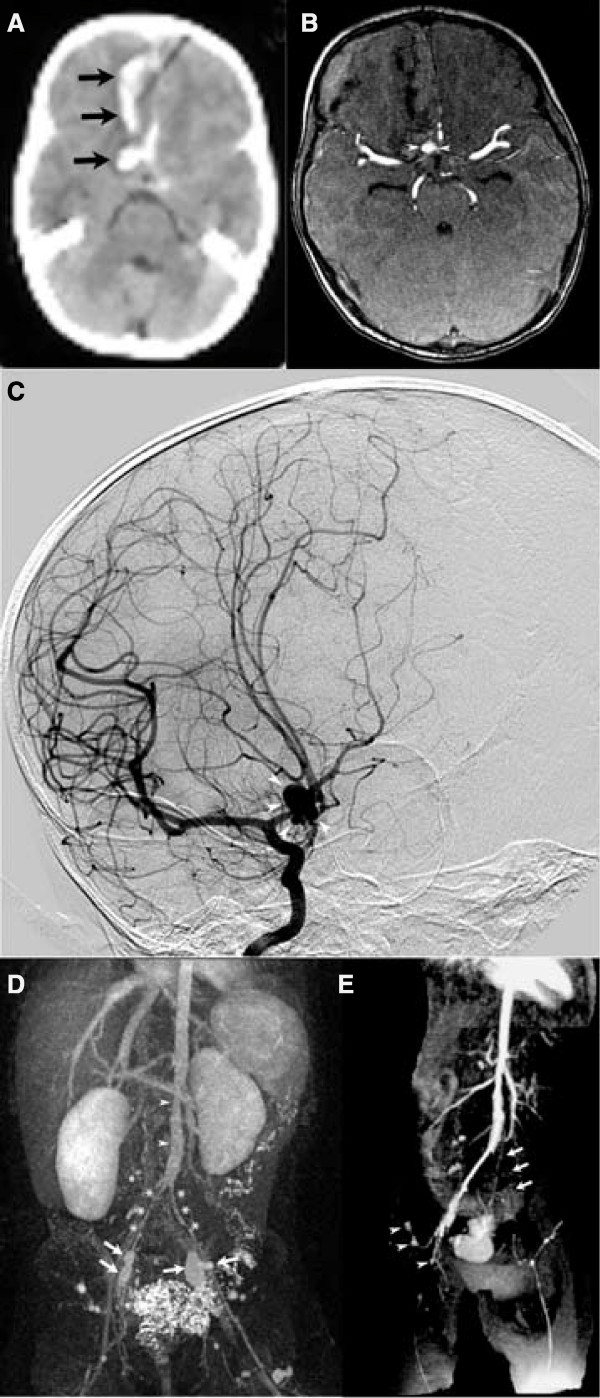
Non-contrast head CT (Fig 1A) demonstrates frontal lobe hemorrhage (arrows). Source image from MRA of the brain (Fig 1B) demonstrates 8 mm aneurysm in the distal A1 segment of the right ACA (arrowheads). DSA (AP/oblique) from right ICA injections (Fig 1C) confirms aneurysm of the distal A1 ACA segment. 3-D TOF MRA abdomen (Fig 1D) with bilateral internal iliac artery aneurysms (arrows), narrowing of mid-abdominal aorta (arrowheads), diffusely narrowed bilateral common and external iliac arteries (asterisks). 2-D TOF MRA abdomen (Fig 1E) shows worsening involvement of left common iliac artery (arrows), with right external and internal iliac arterial aneurysms unchanged (arrowheads).
